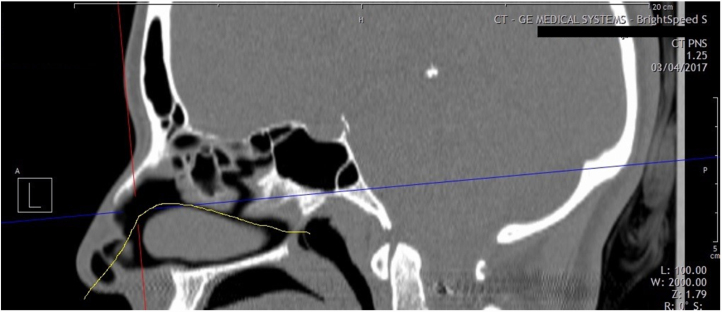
Sagittal reformatted computed tomographic view of nasal cavity. The yellow line indicates the estimated acoustic axis and physiologic nasal airflow.
